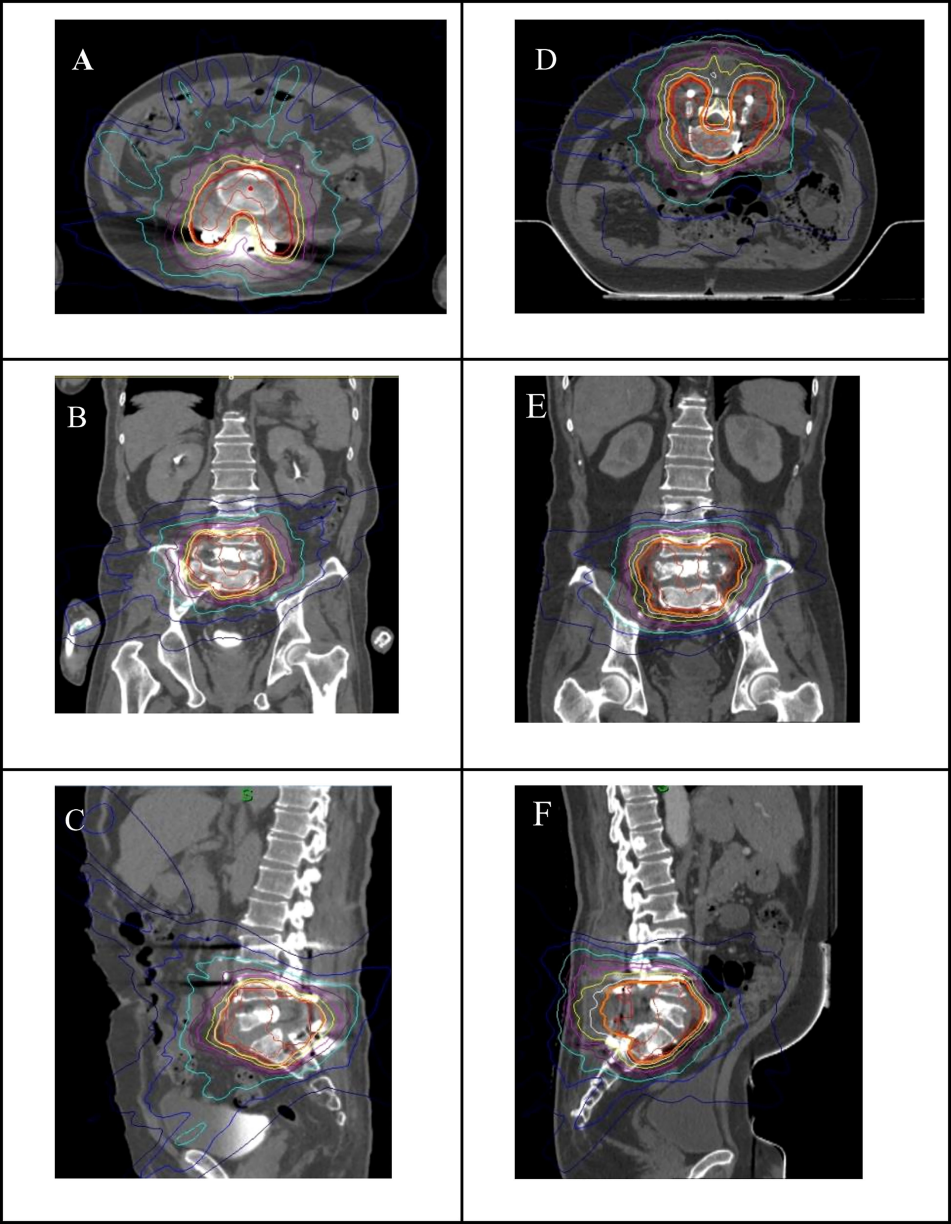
Dose distribution of axial (A), coral (B) and sagittal (C) CT images in supine position, and dose distribution of axial (D), coronal (E) and sagittal (F) CT images in prone position.
